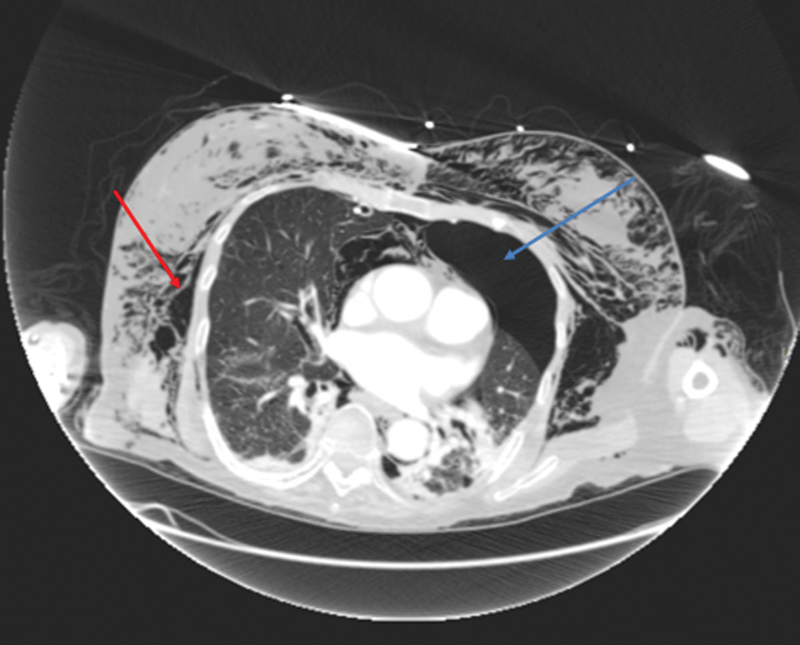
Axial computed tomography image of extensive subcutaneous emphysema in the thoracic wall (red arrow) and left pneumothorax (blue arrow).
